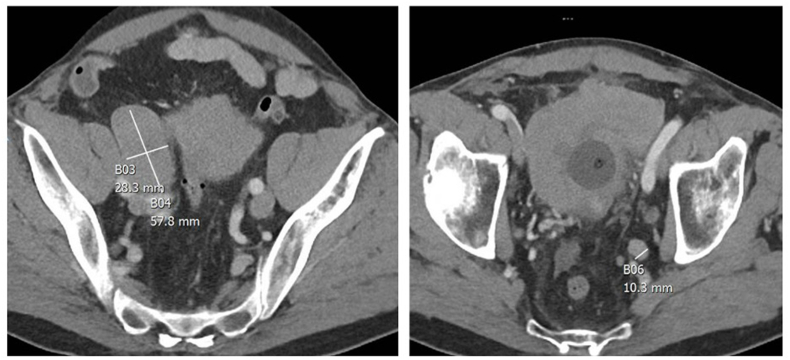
CT chest abdomen pelvis with contrast prior to systemic therapy. Left: bulky pelvic lymphadenopathy with 6cm lymph node at right external iliac. Right: Left lymphadenopathy in obturator fossa and bulky bladder disease.
